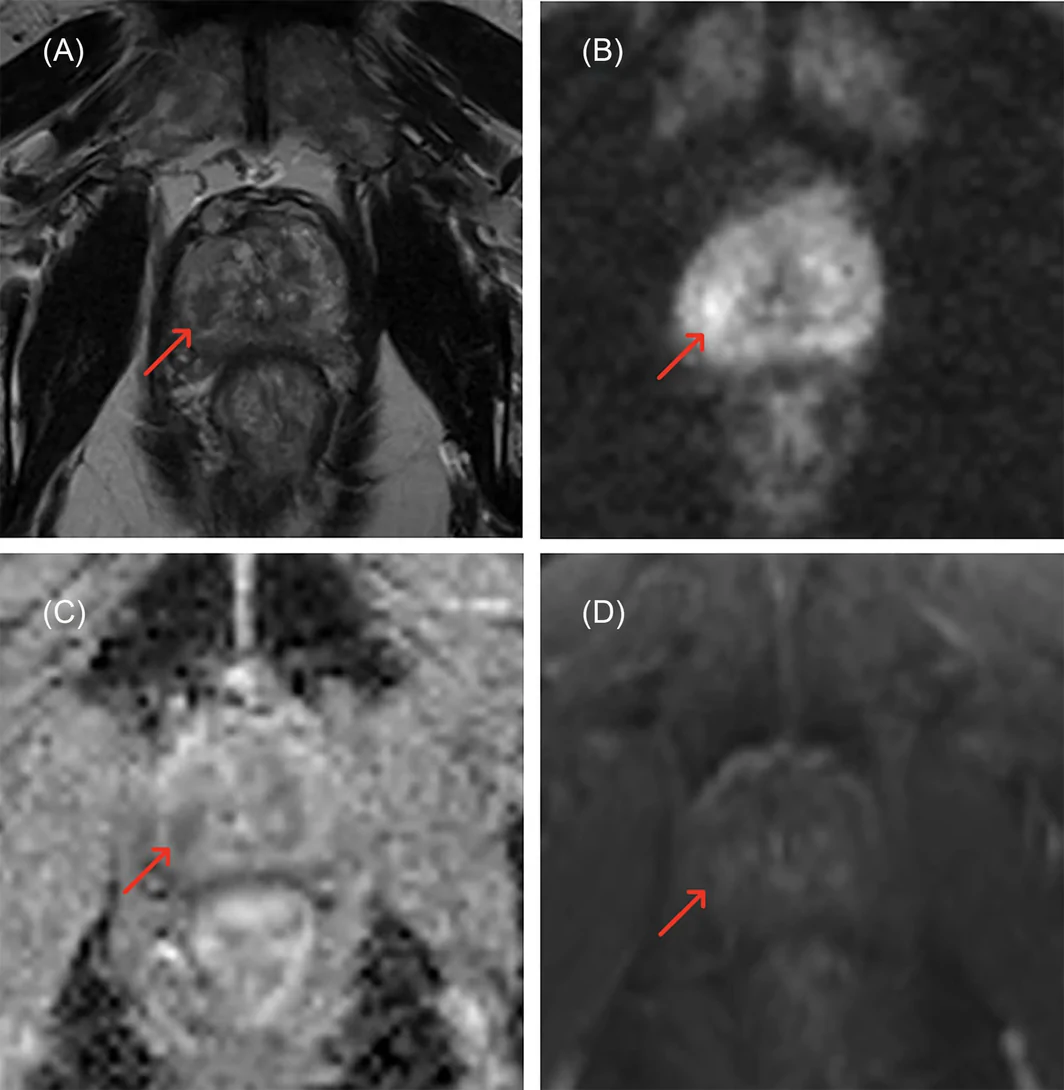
Multiparametric MRI of a PI‐RADS 4 lesion in the right apex peripheral zone: On the axial T2‐weighted image (A), the lesion shows mildly hypointense signal, and on the DWI phase (B), the lesion is moderately hyperintense. The corresponding ADC map (C) shows mild hypointensity, consistent with a PI‐RADS 3 assessment. However, the lesion demonstrates early focal enhancement on the DCE phase (D), meeting criteria for a positive DCE. According to PI‐RADS v2.1, the lesion is therefore upgraded to PI‐RADS 4.
